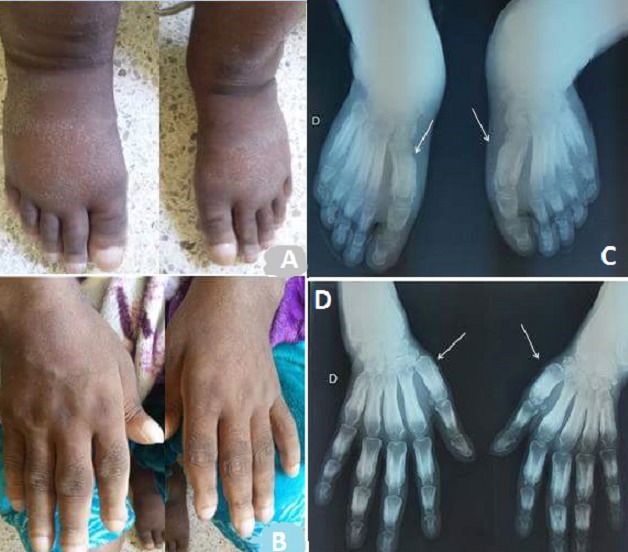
(A, B) un syndrome acromélique des mains et des pieds: comportant un hippocratisme digital, une hypertrophie des parties molles et des troubles vasomoteurs (œdème important avec cyanose); (C, D) les radiographies standards des mains et des avant pieds: une apposition périostée lamellaire des phalanges proximales avec infiltration importante des parties molles
